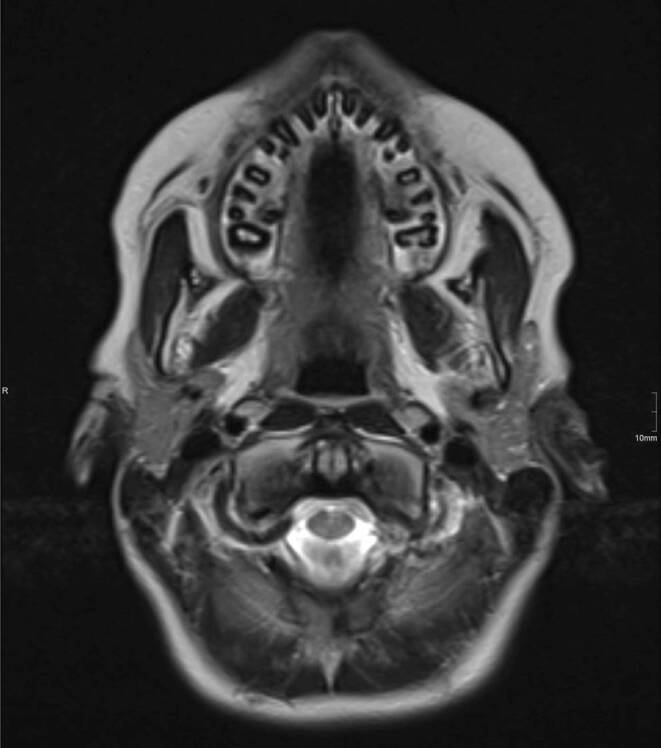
Patient B: MRI image (1.5 T): transversal section at the level of the *dens axis*, T2-weighted TSE sequence (TR: 5.400, TE: 125). Ceramic brackets on the anterior teeth and premolars, metal brackets on the molars, made of the metastable austenitic steel grades 1.4301, 1.4404 and 1.4501 (Dentaurum GmbH & Co. KG). Measured permeability µ_r_ = 1.001. Artifact-free imaging. The alveoli of the maxillary teeth fitted with brackets are also displayed without interference. (Image source: Dr. Gabriele Hahn, Dresden University Hospital) Patient B: MRT-Bild (1,5 T): transversaler Schnitt auf Höhe des *Dens axis*, T2 gewichtete TSE-Sequenz (TR: 5.400, TE: 125). Keramikbrackets an den Frontzähnen und Prämolaren, Metallbrackets an den Molaren, hergestellt aus den metastabilen austenitischen Stahlsorten 1.4301, 1.4404 und 1.4501 (Dentaurum GmbH & Co. KG). Gemessene Permeabilität µ_r_ = 1,001. Artefaktfreie Bildgebung. Auch die Alveolen der mit Brackets versehenen Oberkieferzähne sind störungsfrei dargestellt. (Bildquelle: Dr. Gabriele Hahn, Universitätsklinikum Dresden)
